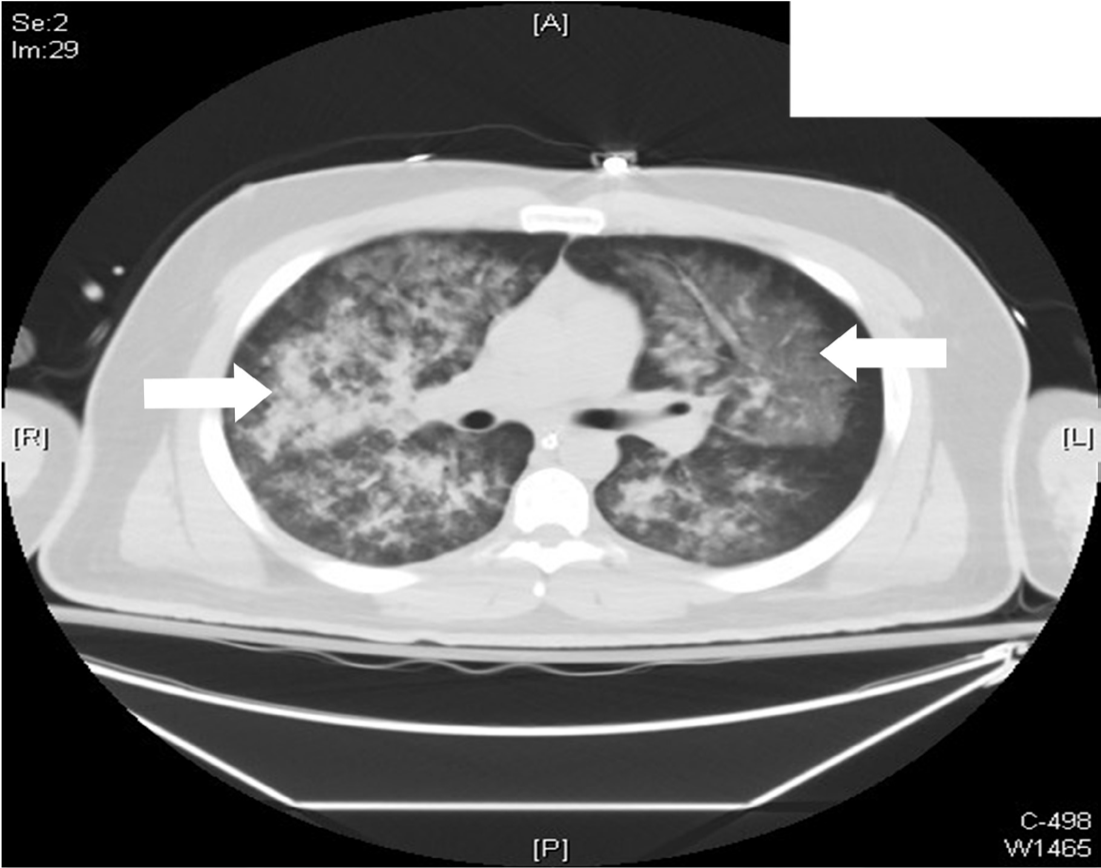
Non-contrast enhanced computed tomography of the chest showing diffuse infiltrates located centrally. White arrows showing infiltrates.
